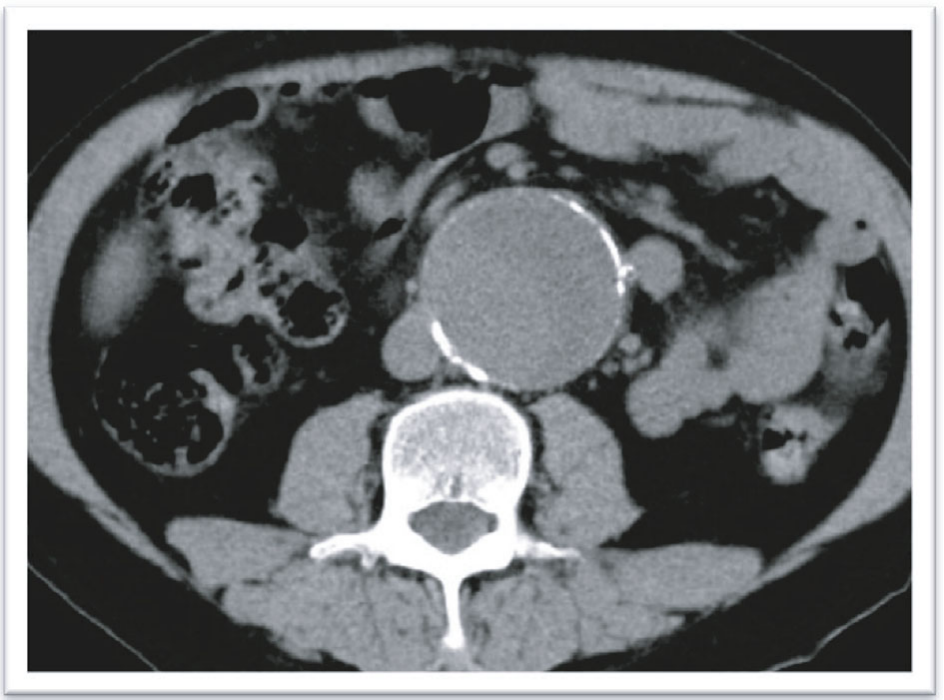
Abdominopelvic CT scan without contrast before AAA repair operation.
